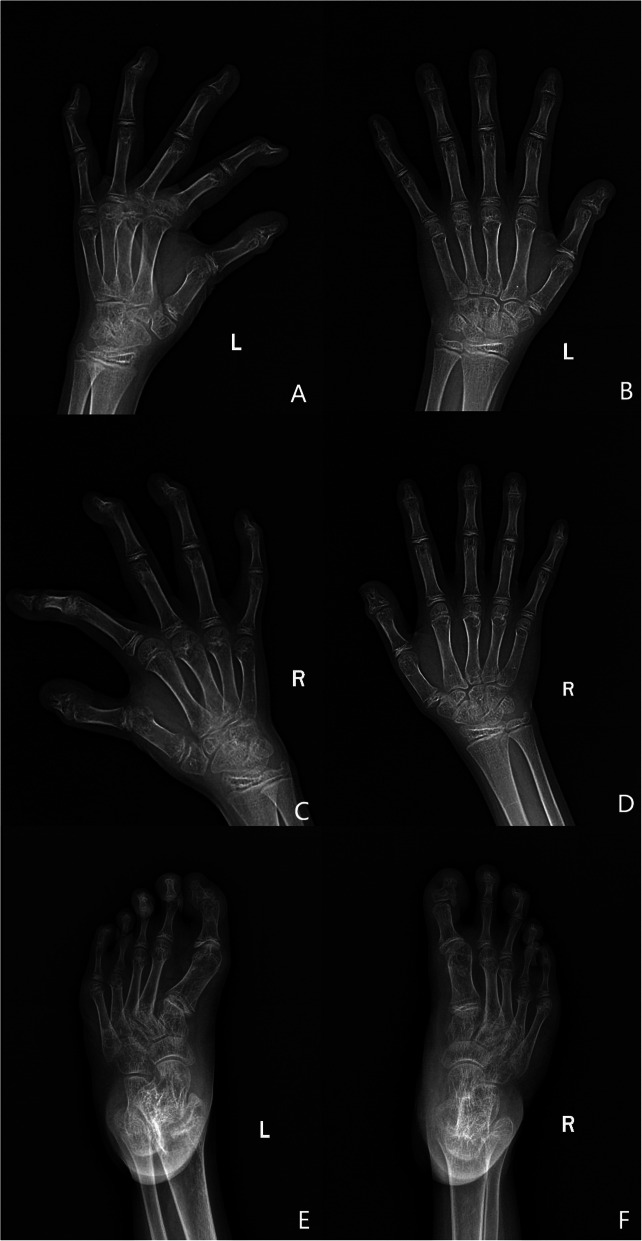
Radiographs of the hands and feet of child 3 aged 8 years. a and b: Left hand, dislocation and advanced carpal bone ages could be observed; c and d: Right hand, dislocation, bifurcation of the distal phalanx of the first finger and advanced carpal bone ages could be observed; e and f: Feet, dislocation could be observed
